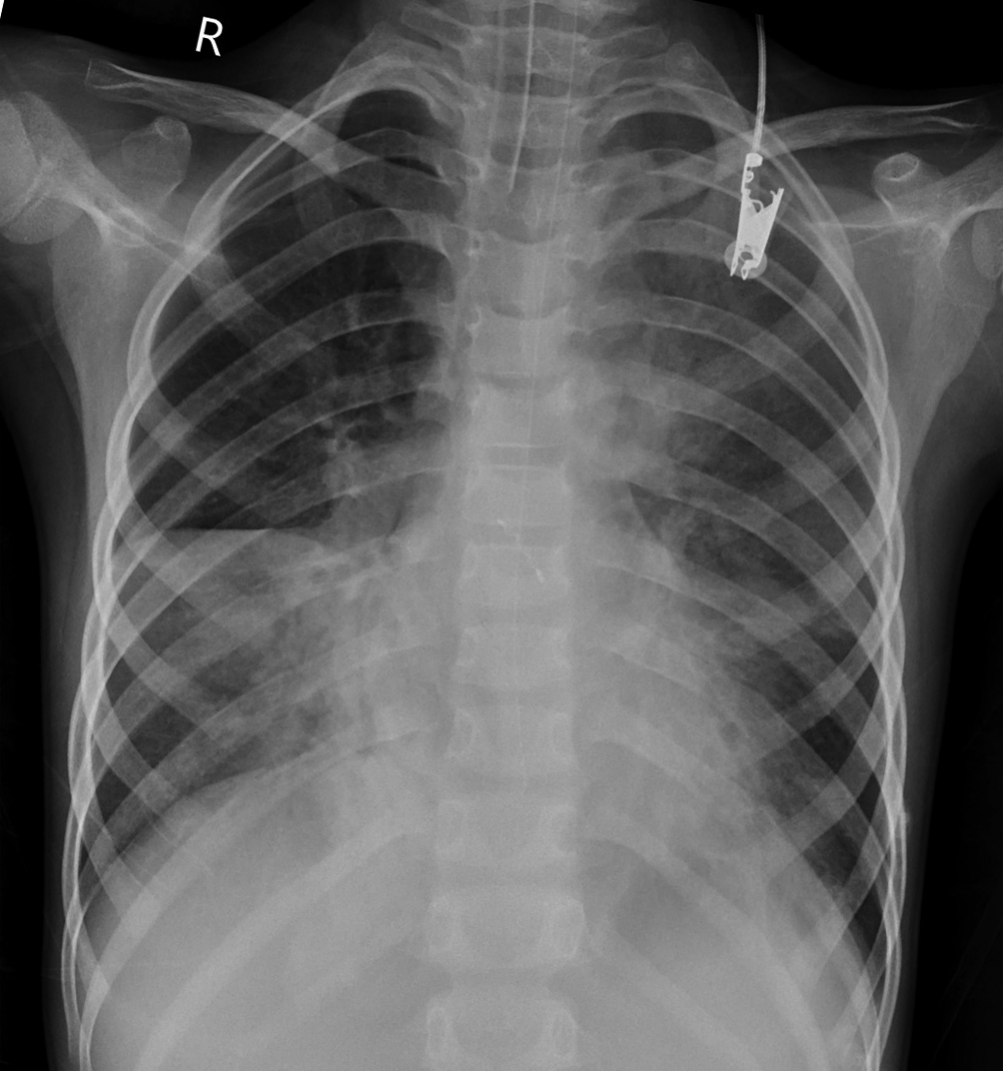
Chest x-ray performed 42 h after ECMO withdrawal.
